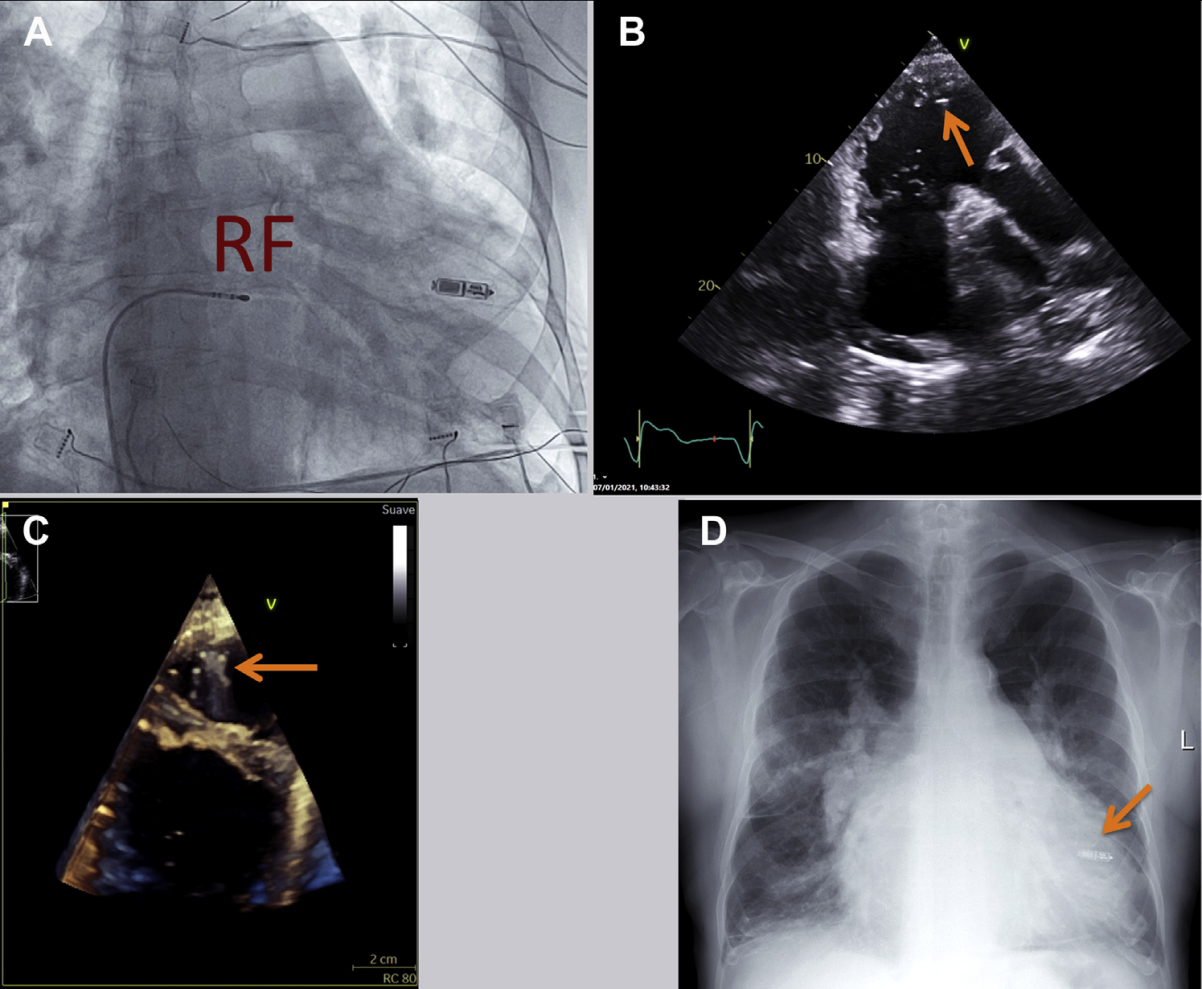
A: Fluoroscopic right anterior oblique view during atrioventricular node ablation after leadless pacemaker (LPM) implantation. *Arrow* indicates position of LPM. RF = ablation catheter. B: Echocardiography modified right 3-chamber view after LPM implantation. *Arrow* indicates position of LPM. C: Three-dimensional echocardiography, right ventricle view. *Arrow* indicates LPM position with identification of 4 tines. D: Posteroanterior thoracic radiograph after LPM implantation in midseptal location. *Arrow* indicates position of LPM.
